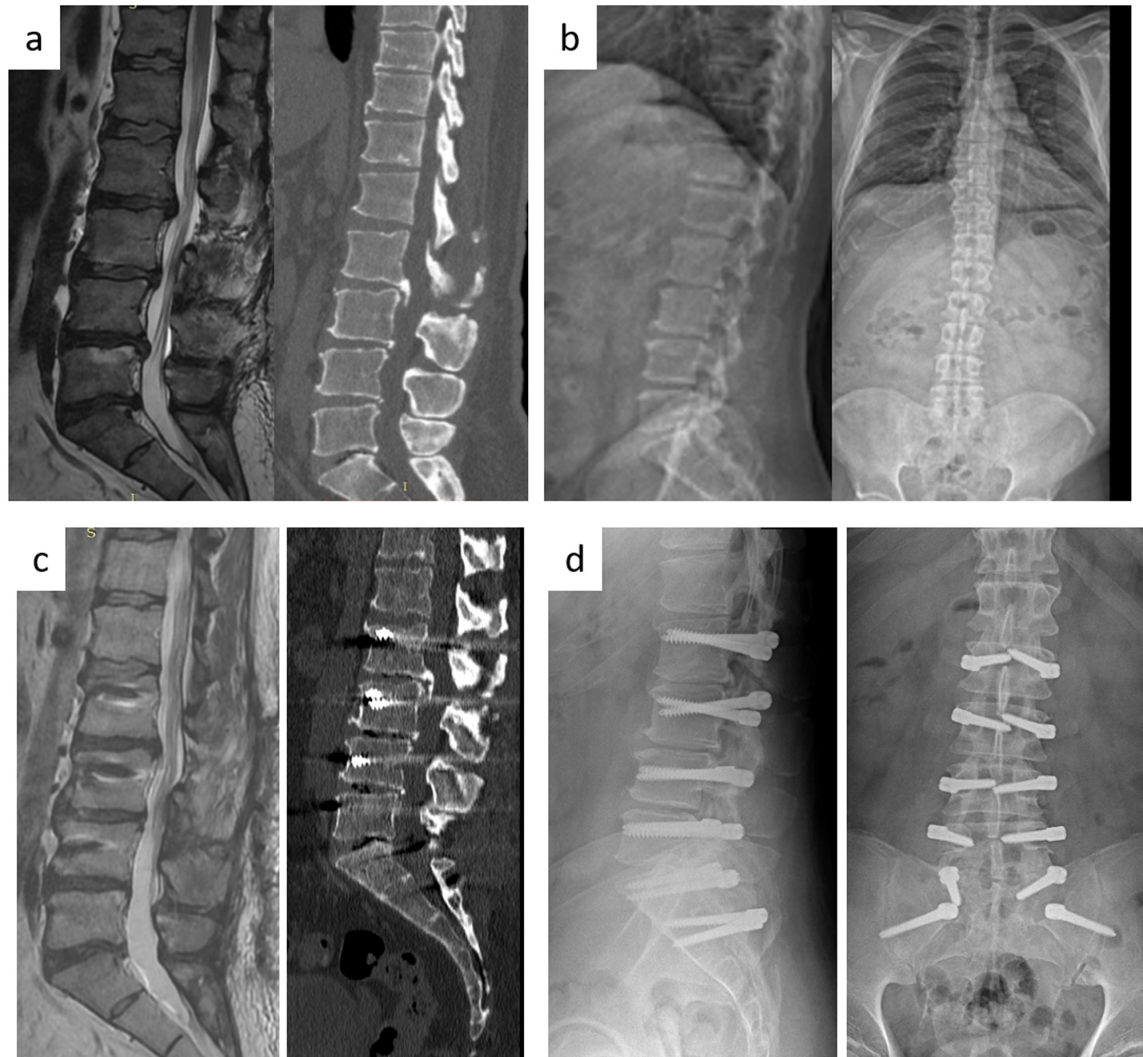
Patient sample a) T2-weighted MR image and CT scan show severe degenerative disc disease in L2-3, L3-4, L4-5 and L5-S1 levels. b) Direct X-ray shows decreased disc height, osteophytes and flat back deformity but no apparent balance problem. c) T2-weighted MR image shows repeated posterior annuluses in every level via microsurgical way and CT scan lateral view shows stabilisation with dynamic system including iliac wings. d) Anterior-posterior (AP) and lateral X-ray.
